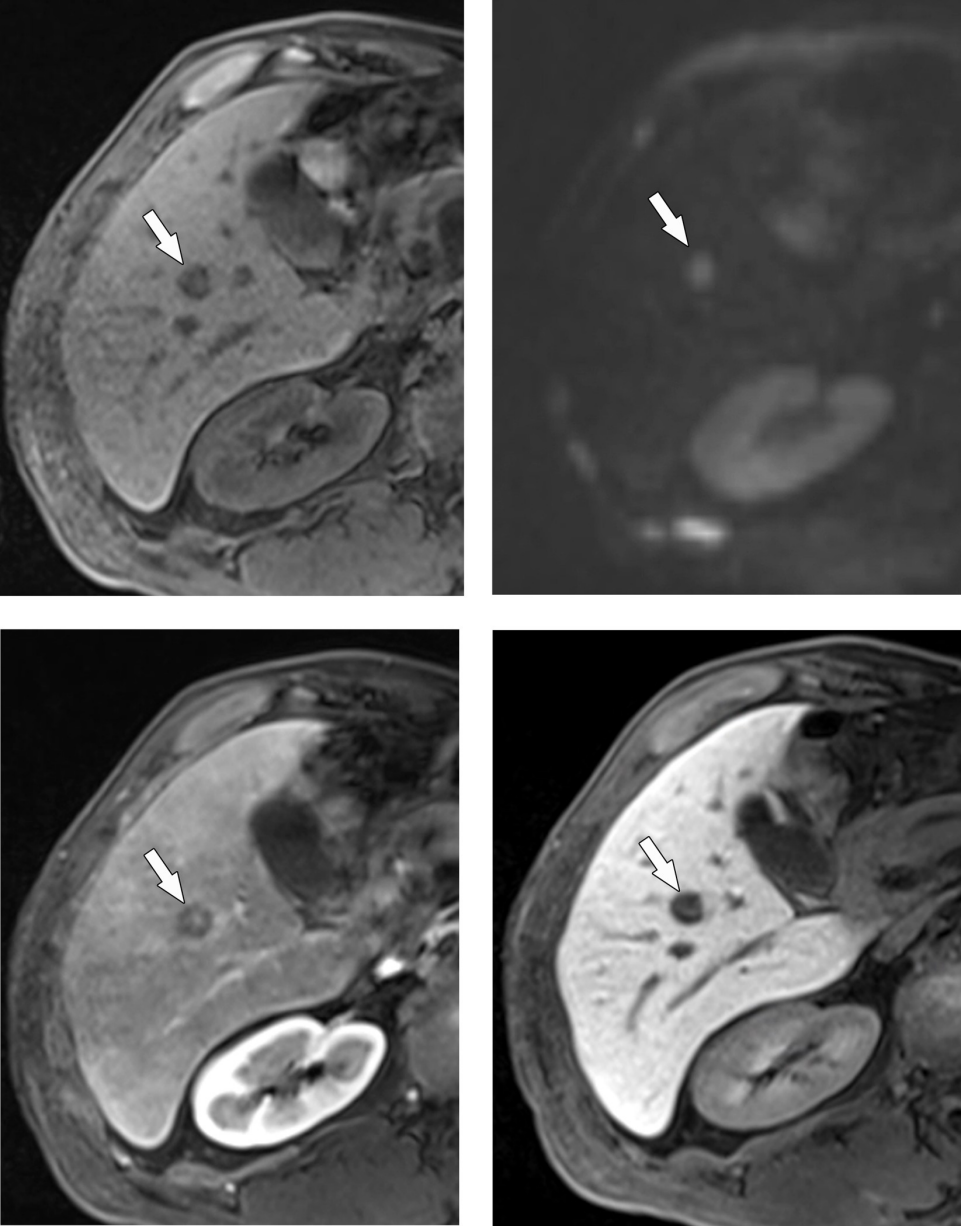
Gadoxetic acid-enhanced MRI in a 59-year-old man with a liver metastasis from non-ocular (oral mucosal) melanoma. Axial gadoxetic acid-enhanced MR images show a single metastasis in the right inferior liver (arrows). It shows low signal intensity on a precontrast T1-weighted image (A), high signal intensity on a diffusion-weighted image (b = 800 s/mm^2^) (B), low signal intensity with peripheral enhancement on a portal phase T1-weighted image (C), and low signal intensity on the hepatobiliary phase (D), compared to the surrounding liver.
